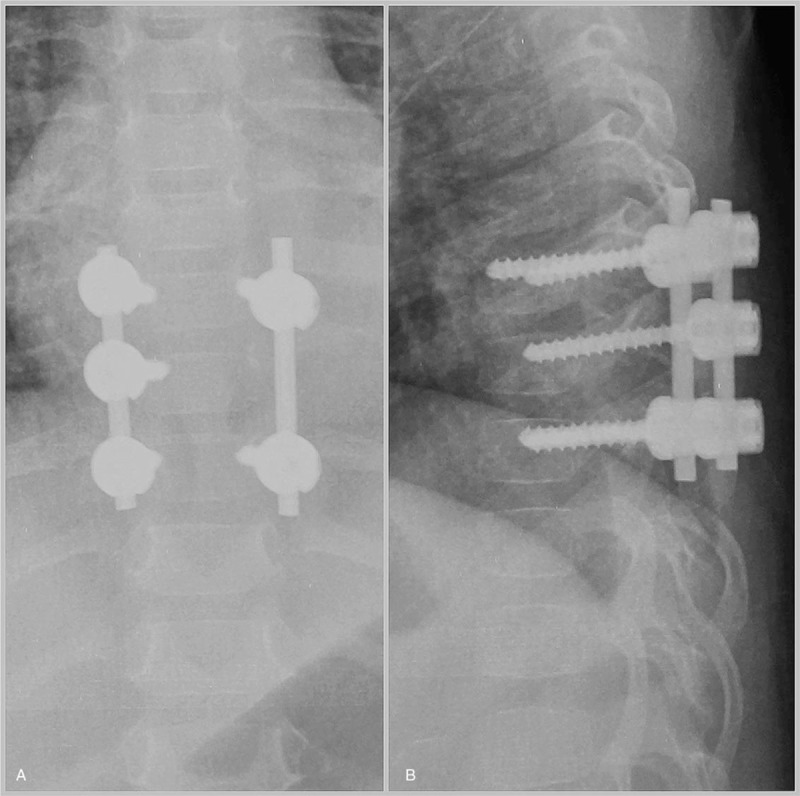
(A) Posteroanterior X-ray image of the thoracic spine obtained postoperatively. (B) Lateral X-ray image of the thoracic spine obtained postoperatively.
